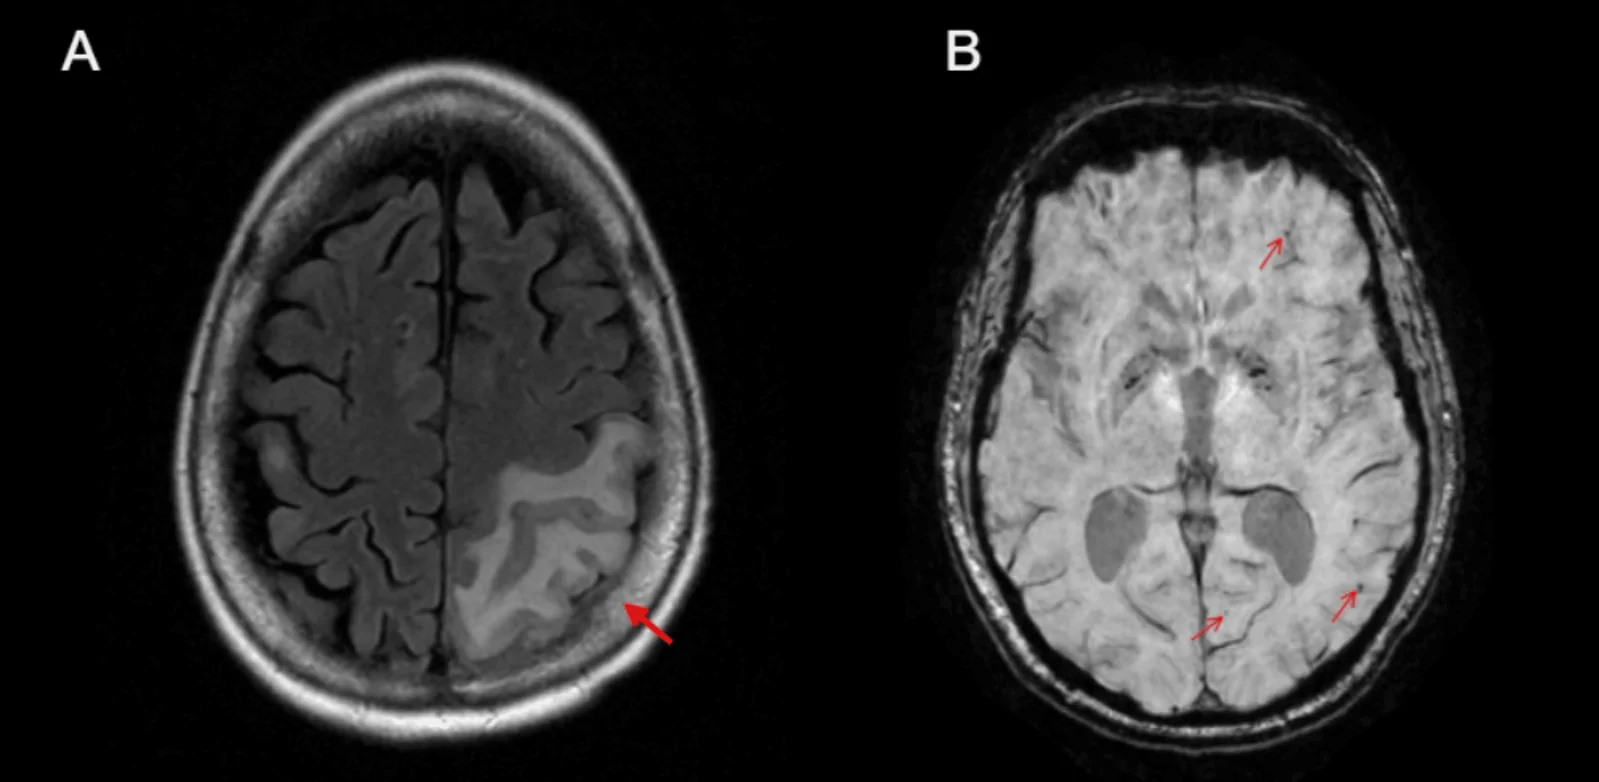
Representative ARIA-E and ARIA-H findings in patients treated with lecanemab. Representative amyloid-related imaging abnormalities (ARIA) in two lecanemab-treated patients. (A) Axial fluid-attenuated inversion recovery (FLAIR) image in a 76-year-old woman shows left parietal vasogenic edema/sulcal effusion (arrow), consistent with ARIA-E. (B) Axial susceptibility-weighted imaging (SWI) image in a 77-year-old man shows multiple punctate susceptibility foci (arrows), consistent with ARIA-H microhemorrhages.
